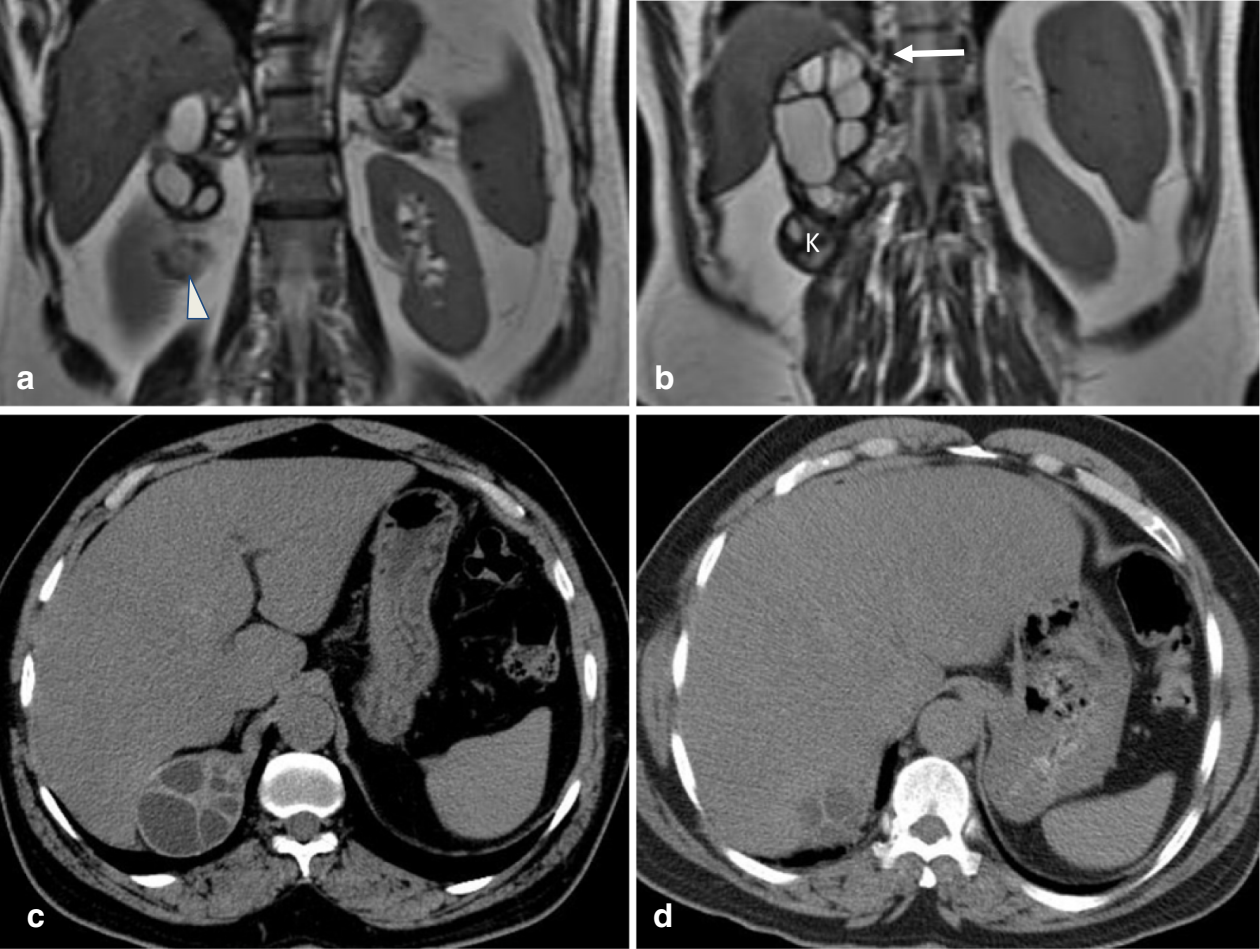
T2-weighted magnetic resonant coronal images (a, b) and computed tomography axial images (c, d) show a multiloculated cystic lesion in the retroperitoneum. This abuts the lower surface of the liver and the right crus of the diaphragm (thick white arrow) as well as the posteromedial aspect of the dome. It also abuts the right kidney which is partially visualized (k). A smaller lesion within the kidney is also seen (arrowhead). Note that the dome of the diaphragm is intact on this scan
